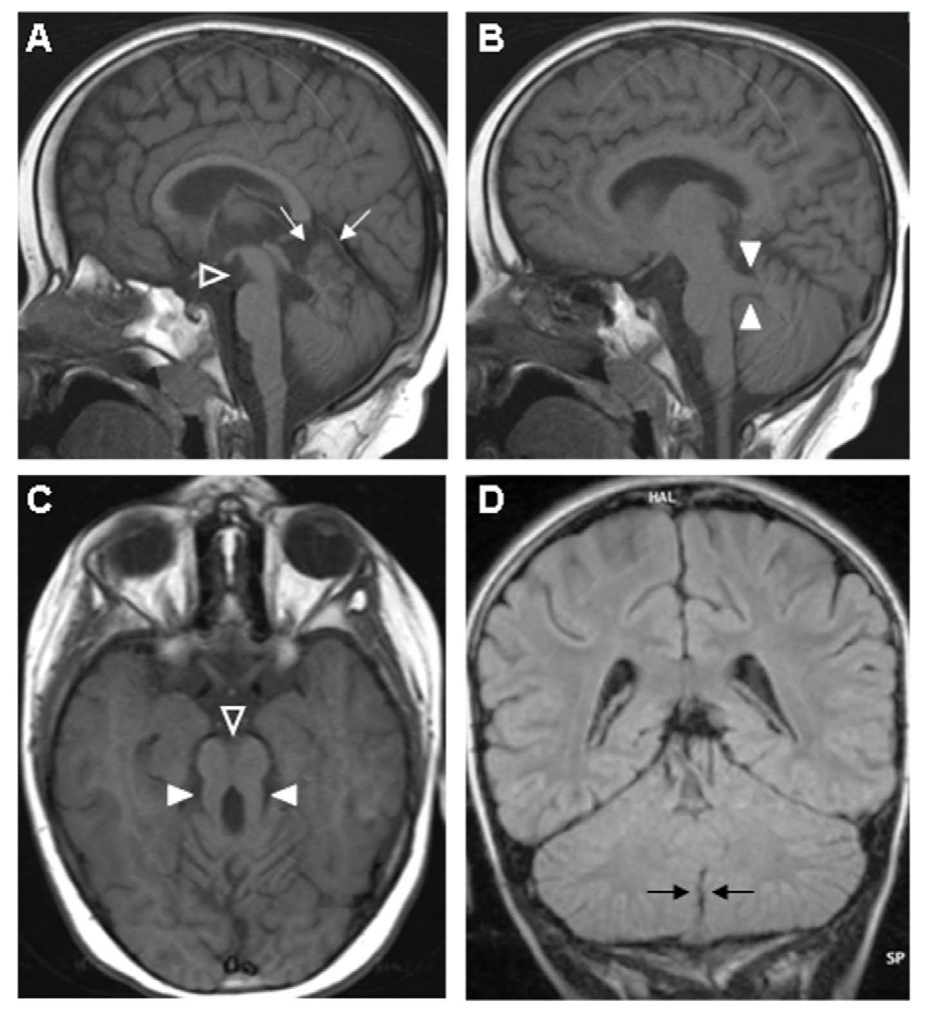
Brain MRI sections in patients with JSRD. A) mid-sagittal T1-weighted image shows a thin midbrain with corresponding enlargement of the interpeduncular fossa (open arrowhead). There is concurrent superior vermian dysplasia (thin arrows); B) parasagittal T1-weighted image shows thickened and maloriented superior cerebellar peduncle (thick arrowheads); C) axial T1-weighted image confirms the deepened interpeduncular fossa (open arrowhead) and abnormal superior cerebellar peduncles (thick arrowheads), comprising the "molar tooth sign"; D) coronal FLAIR image shows midline cerebellar cleft (black arrows) indicating agenesis of the inferior vermis.
